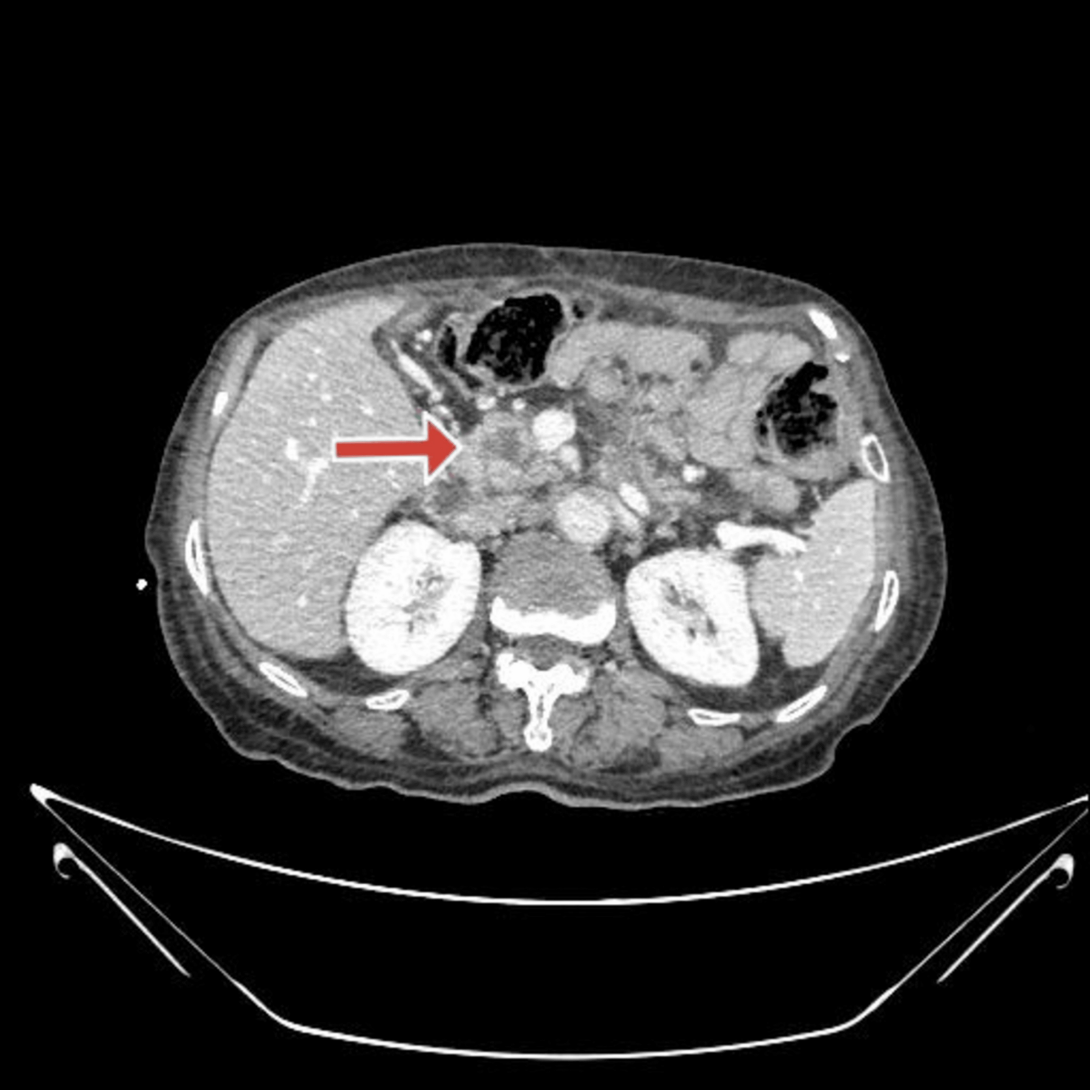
The CT scan of the patient showing a 2 cm lesion in the pancreatic head.
